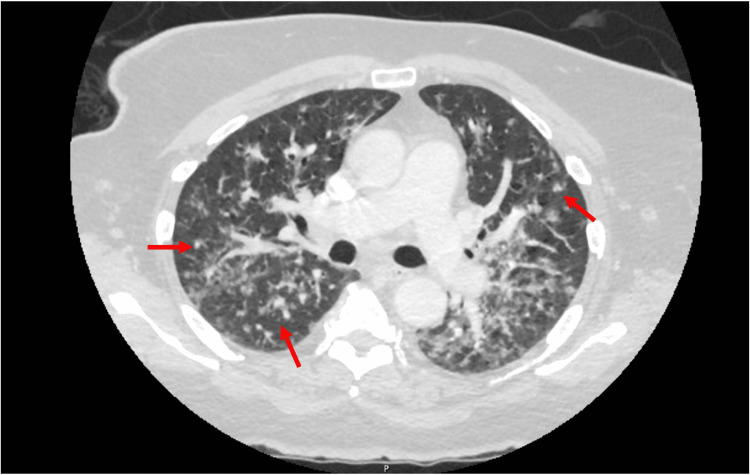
CT chest, abdomen, and pelvis showing multiple subcentimeter pulmonary nodules measuring up to 5mm (arrows) with diffuse tree-in-bud nodularity throughout the lung parenchyma.
